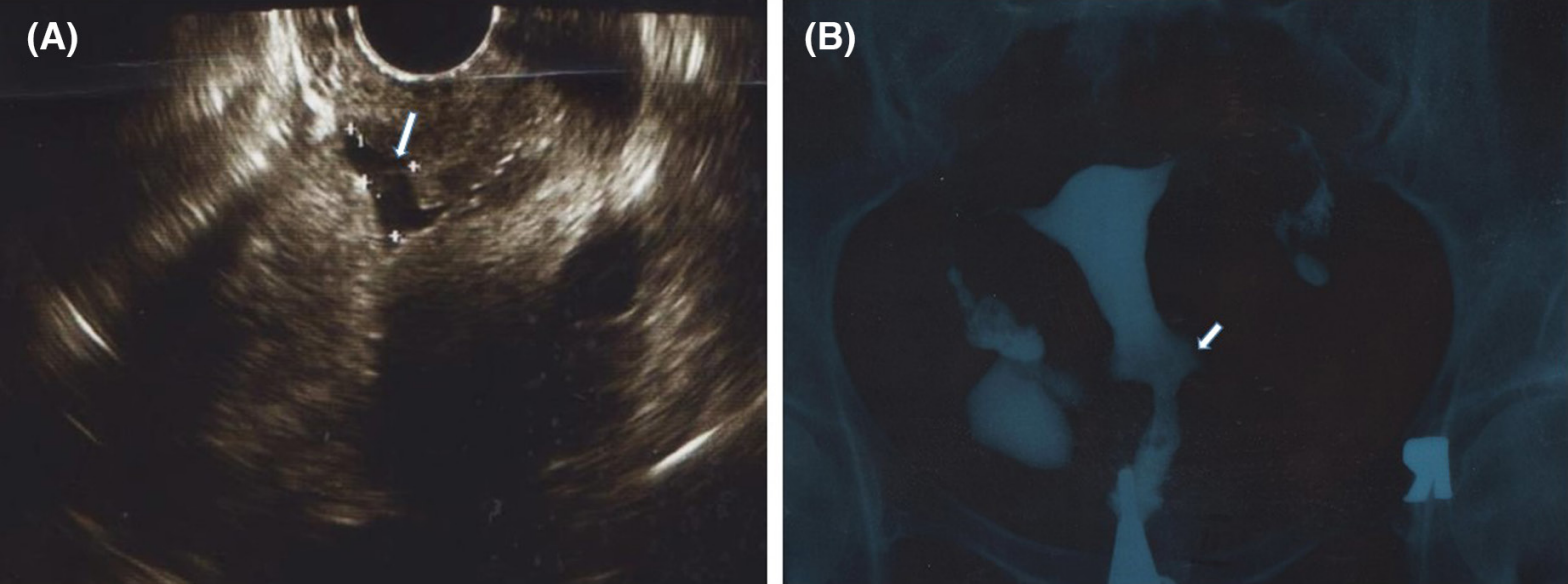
Isthmocele in the patient's uterine (A) The white arrow shows isthmocele on hysterosonography (B) The white arrow points to isthmocele's pouch on HSG
